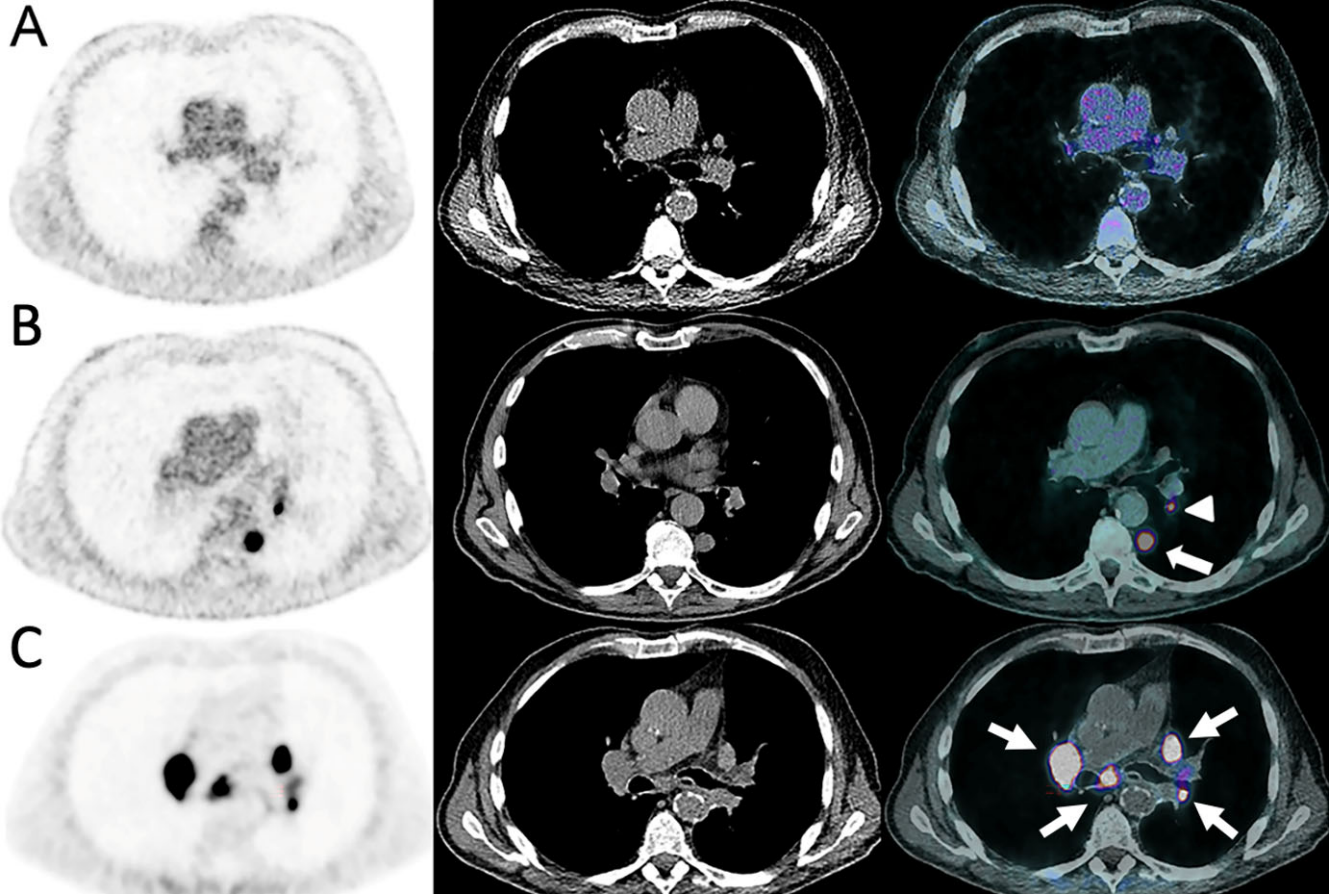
Sarcoidosis-like reaction in an 84-year-old male with cutaneous melanoma of unknown primary. The disease initially presented as the incidental discovery of a lung nodule on CT (A, B). A^18^F-FDG-PET/CT was done and showed a solitary left pulmonary lower lobe nodule (arrow) with a homolateral hilar lymph node (arrowhead) which were both highly metabolic. Mediastinum and upper hilum were disease free. The patient underwent upfront surgery which established the diagnosis. Nivolumab was administered 1 month post-surgery and after 4 cycles the patient developed dyspnea. (C) A^68^Ga-DOTATOC-PET/CT showed intense and diffuse uptake in enlarged mediastino-hilar lymph nodes (arrows) suggesting a reactive inflammatory process with high uptake of activated lymphocytes strongly expressing STTR-2 targeted by ^68^Ga-DOTATOC.
